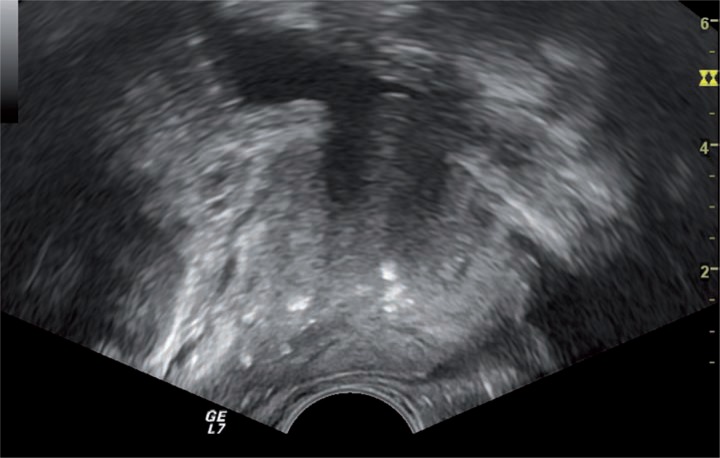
Transrectal ultrasound in longitudinal section of prostate shows mild calcifications with 1 or multiple small foci without coarse shadow.
